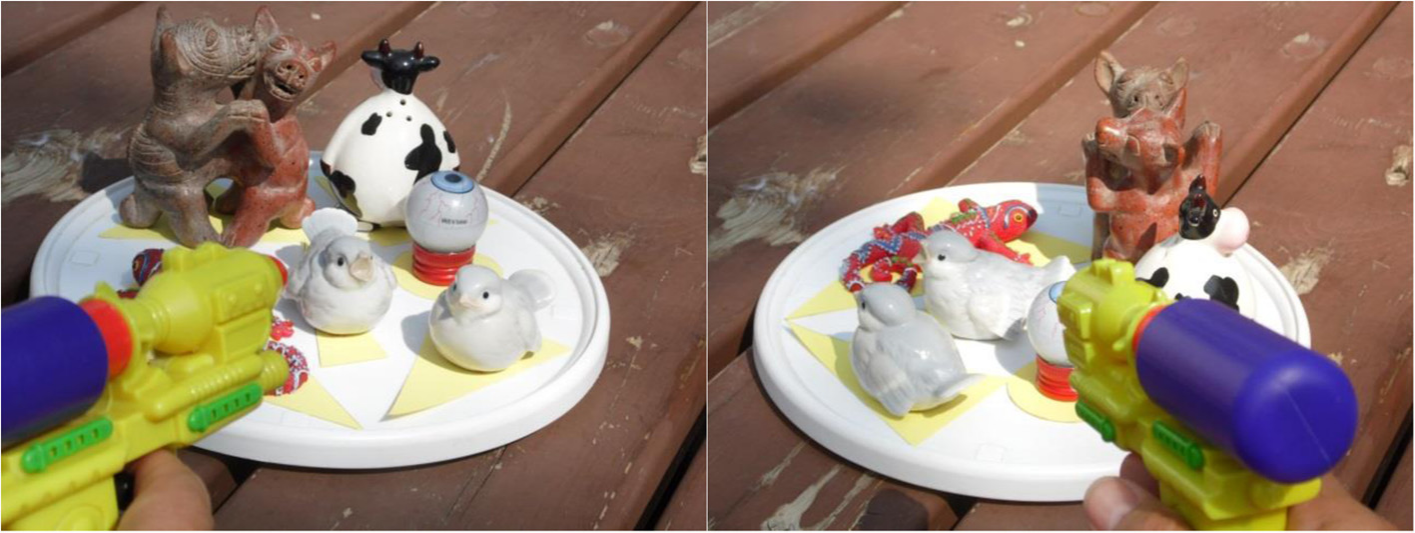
A mockup of what the screen might look like in a CancerZap! video game. The player shoots x-ray photons at a scene that is rotating, with one object (the Martian eye [152]) in this case representing the “bad guy” tumor. The gun’s lateral motion would correspond to a fan beam. As in CT Brush, the objects would only become visible as they were shot at, accumulating x-ray dose. In the plane, the ornate figures would be represented by simpler “footprints”, such as triangles and circles, shown here beneath them.
